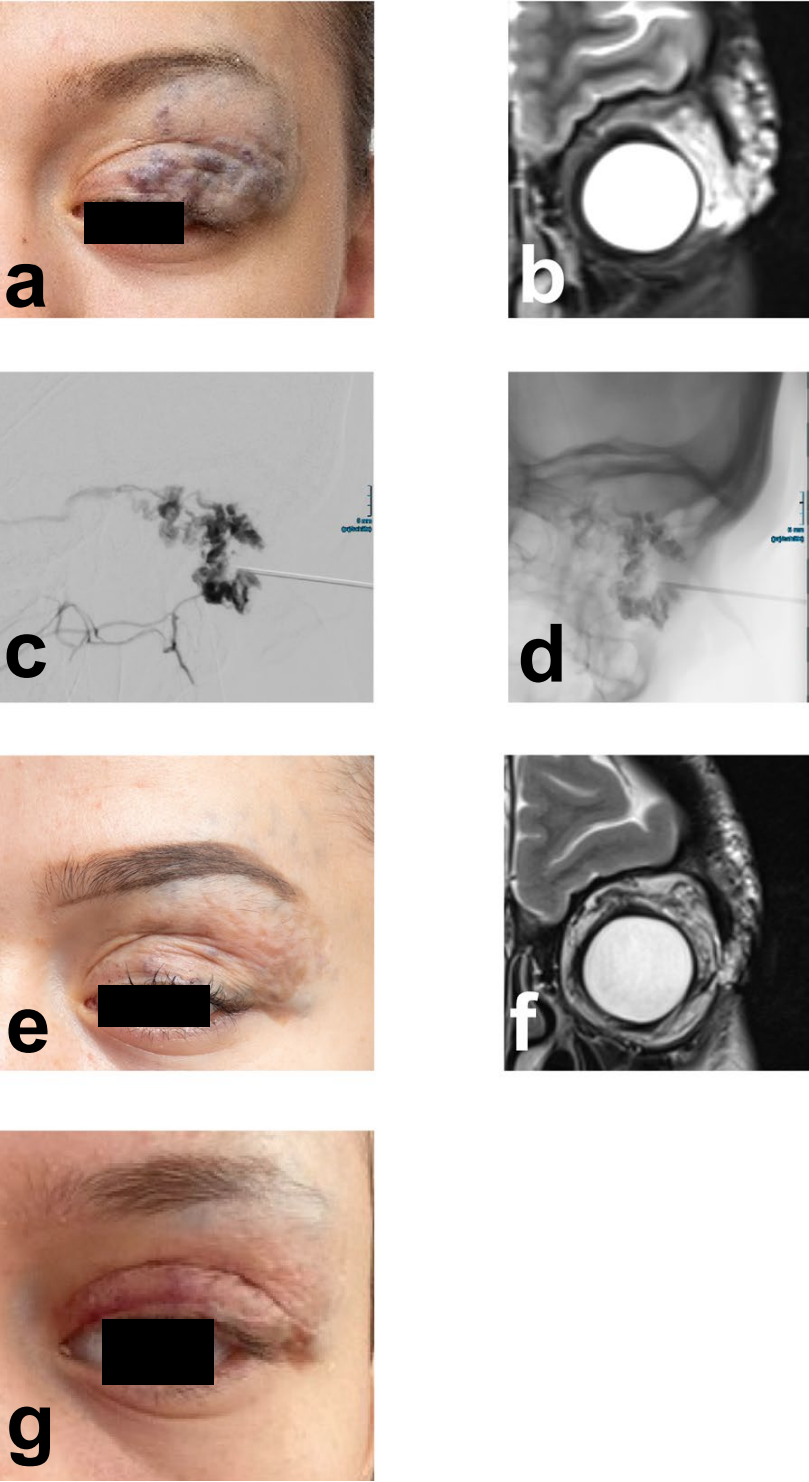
Progressive improvement of venous malformation on the eyelid under BEST therapy. A female patient (age 20 at her first session) underwent treatment with BEST for a vascular malformation of the eyelid. Although the lesion exhibited a relatively modest initial volume (33.5 mL), it resulted in functional impairment and had a marked cosmetic impact, both of which adversely affected the patient’s quality of life. Following three sessions of BEST, the lesion volume was reduced to 5.5 mL, representing an 83.6% reduction. This substantial volumetric reduction led to a substantial clinical improvement alleviating functional deficits and enabling subsequent surgical intervention for further esthetic refinement. a Patient’s condition prior to treatment, highlighting discoloration and swelling and b the corresponding MRI of the lesion. c and d Digital subtraction angiography showing the injection of bleomycin during BEST for the treatment of a lesion on the eyelid. e Final follow-up after 2 years and 4 months, demonstrating a notable reduction in lesion volume and swelling (f) as seen on MRI. The clinical photograph also highlights smoother skin texture and less discoloration. g Post-procedural image showing the patient after BEST, followed by eyelid correction surgery to restore symmetry
